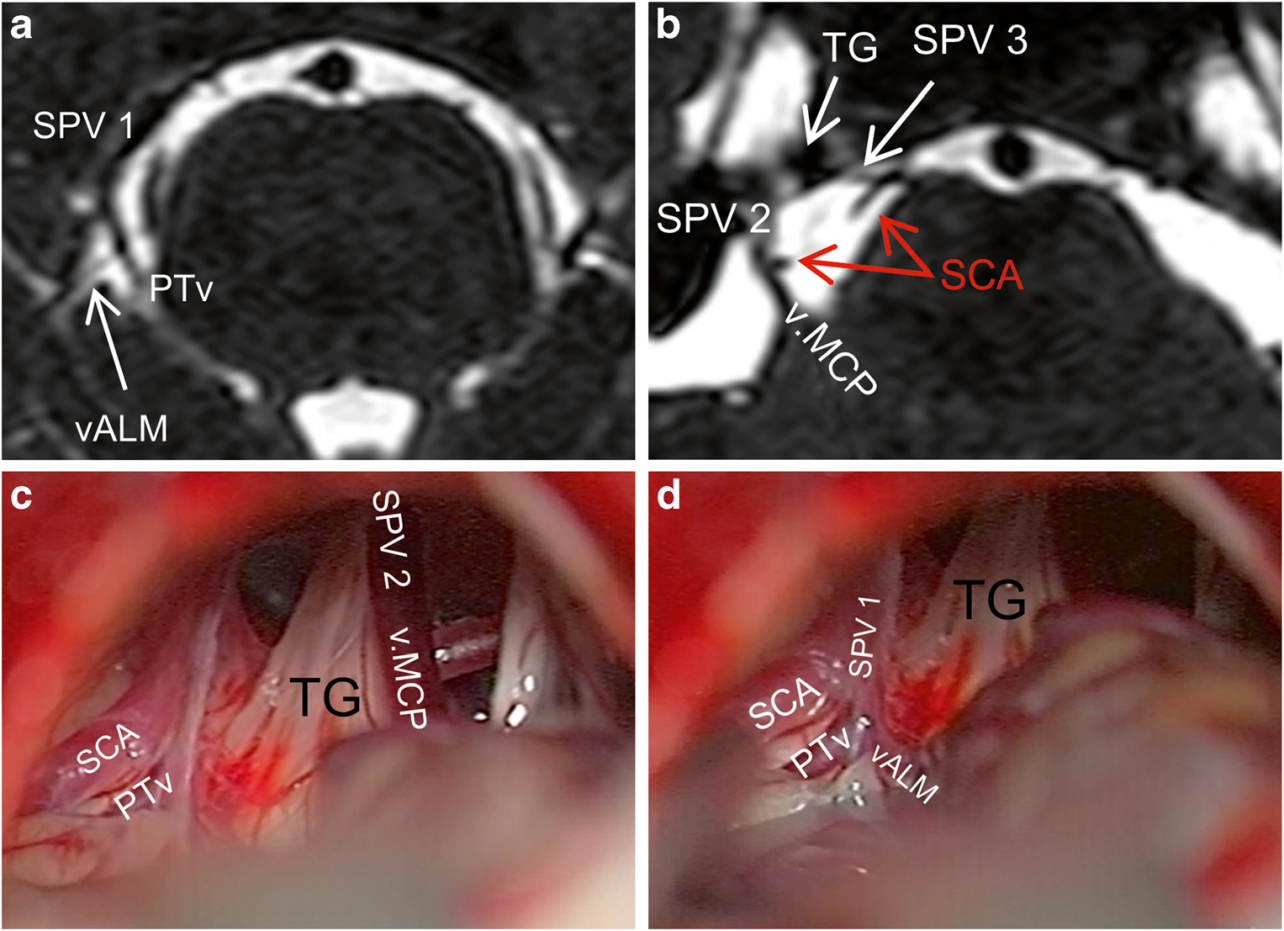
A case of right-sided CPA. a Axial bFFE magnetic resonance imaging showing an SPV1 composed of an ALMv and a PTv. b Another axial section showing an SPV 2 as the continuation of the v.MCP as well as an SPV 3 as a continuation of an TPv. c, d Corresponding intraoperative photographs of the same case. We can see here all the above described SPVC components, except the SPV 3 and its single tributary (TPv). This is because of the very medial course of the TPv and medial type III drainage of the SPV 3 into the SPS beyond the microsurgical field. ALMv anterior lateral marginal vein, bFFE balanced fast field echo, CPA cerebellopontine angle, SCA superior cerebellar artery, SPV superior petrosal vein, SPVC superior petrosal vein complex, SPV1 first SPV, SPV2 second SPV, SPV3 third SPV, TG trigeminal nerve, TPv transverse pontine vein, v.CPF vein of cerebellopontine fissure, v.MCP vein of the middle cerebellar peduncle
